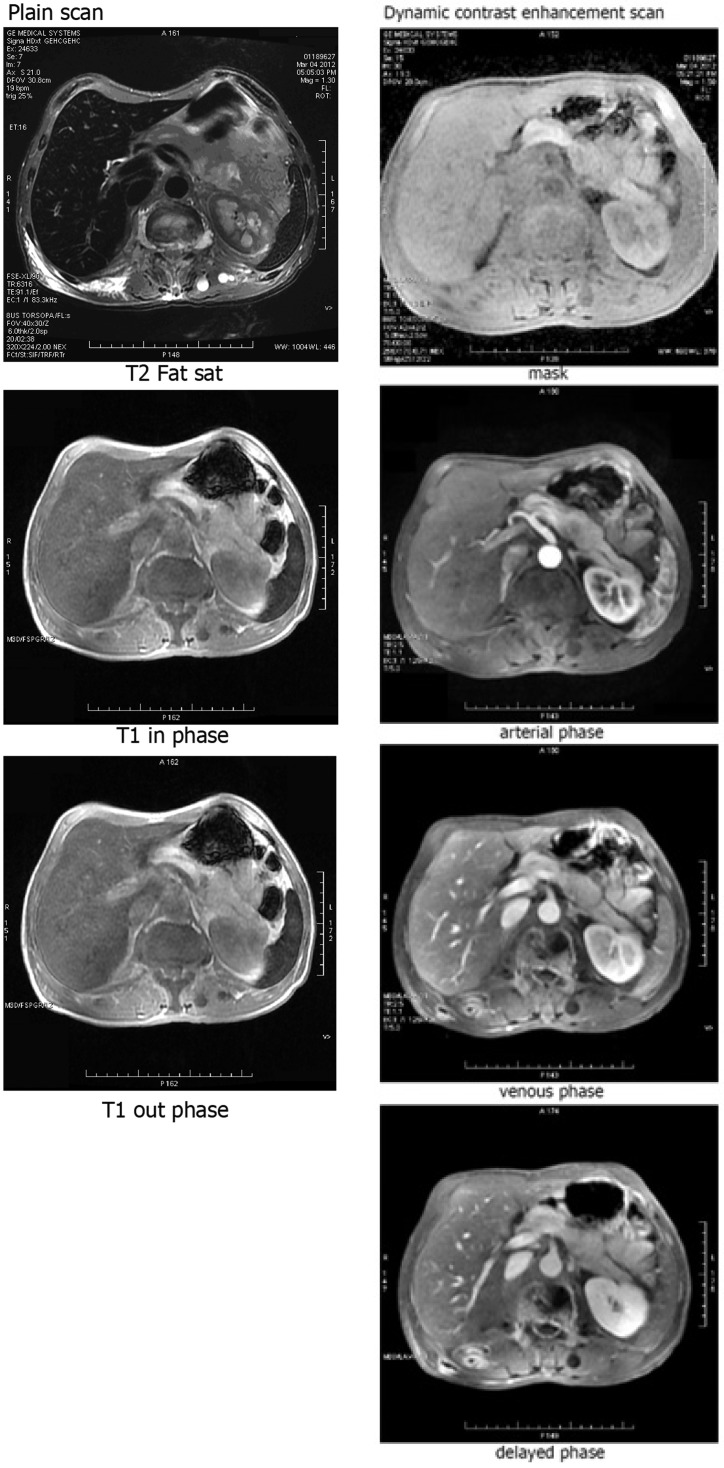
Abdominal magnetic resonance imaging (MRI) scan showing a 2.1×1.8-cm mass with mixed T1 and mixed T2 abnormal signals in the body of the pancreas, with circular-like and peripheral edema. Neighboring tissues were compressed. Enhanced MRI scans showed that the mass was unevenly enhanced.
